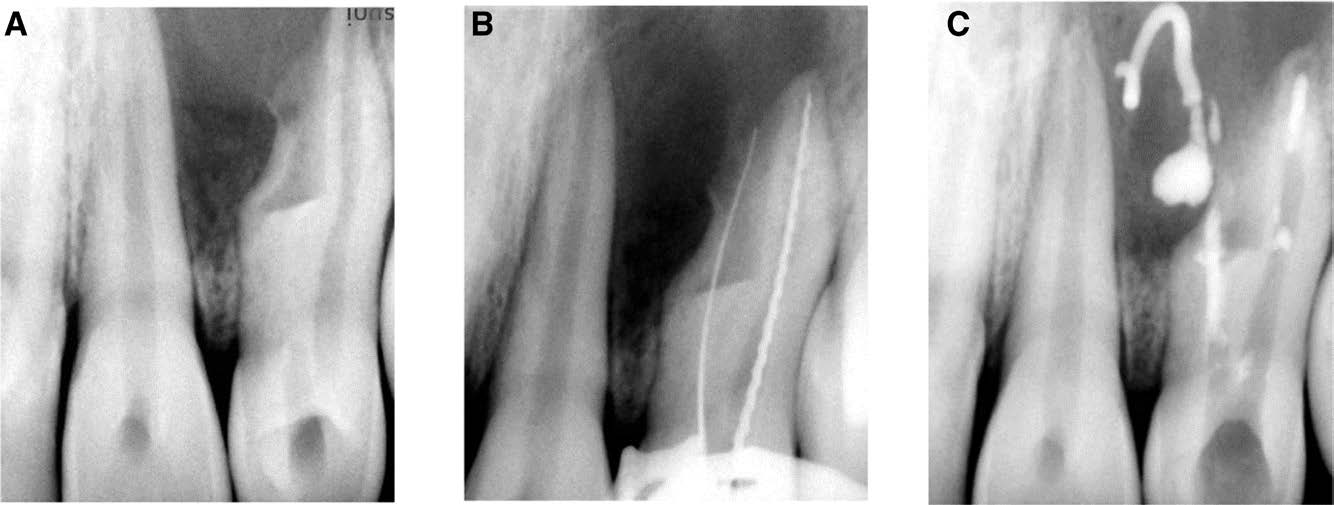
(A) Periapical radiograph of tooth #10 displaying DI Oehler type III, along with wider dimension mesiodistally compared to tooth #9, complex internal anatomy, and periapical radiolucency along with previous access cavities in teeth #9, #10. (B) Periapical radiograph of tooth #10 to confirm the working of the regular root canal on the distal side and the pseudo canal on the mesial side. (C) Inter-appointment application of calcium hydroxide paste in the canals with some overflow on the mesial side of tooth #10. DI = dens invaginatus.
